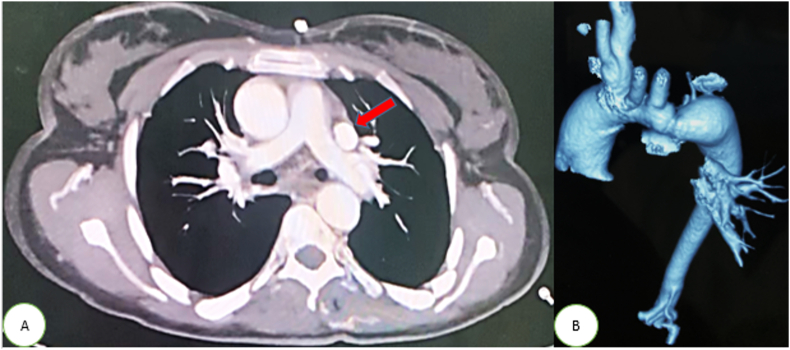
(A) Computed Tomography Chest showing persistent left superior vena cava (Red arrow) (B) 3D reconstruction of Computed tomography image showing an aspect of the narrowed aortic arch with a diameter of 6 mm, measured 9 mm bellow the origin of the left subclavian artery. (For interpretation of the references to colour in this figure legend, the reader is referred to the Web version of this article.)
